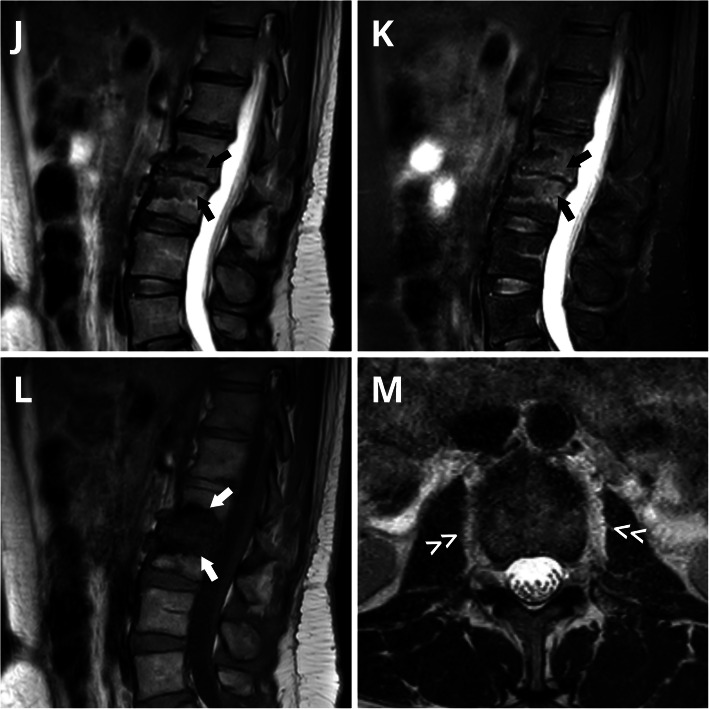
Lumbar MRI performed 2.5 years after the initial diagnosis. The sagittal T2WI (J) and the STIR-WI (K) demonstrate increased areas of hyperintensity in the L2 and L3 vertebral bodies and endplates, indicating progression of the oedema (black arrows). The sagittal T1WI (L) depict the same irregularities and lesions with marked hypointensity of L2–L3 vertebral body endplates (white arrows). The axial T2WI (M) show the progression of the enlarged paravertebral surrounding soft tissues, suggestive of inflammation (double arrows)
